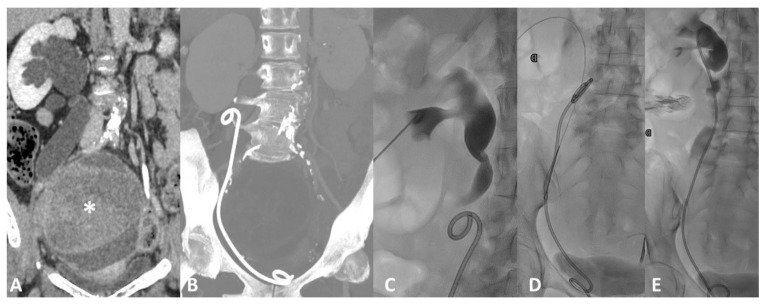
Device migration. Forty-six-year-old female with rapid onset of right flank pain ad diuresis reduction 3 months after ureteral stent placement for marked hydroureteronephrosis (A) caused by an ovarian mass (*). Follow-up CT scan (B) shows displacement of the ureteral stent, slipped down in the urinary tract. Fluoroscopic image (C) shows the consequent dilatation of the renal pelvis and calyces with stasis of contrast medium caused both by the malfunction of the displaced device and by the mechanical obstruction caused by the stent itself inside the ureter. Percutaneous Stent retrieval with a goose neck snare catheter (D) and replacement (E) was the treatment of choice.
